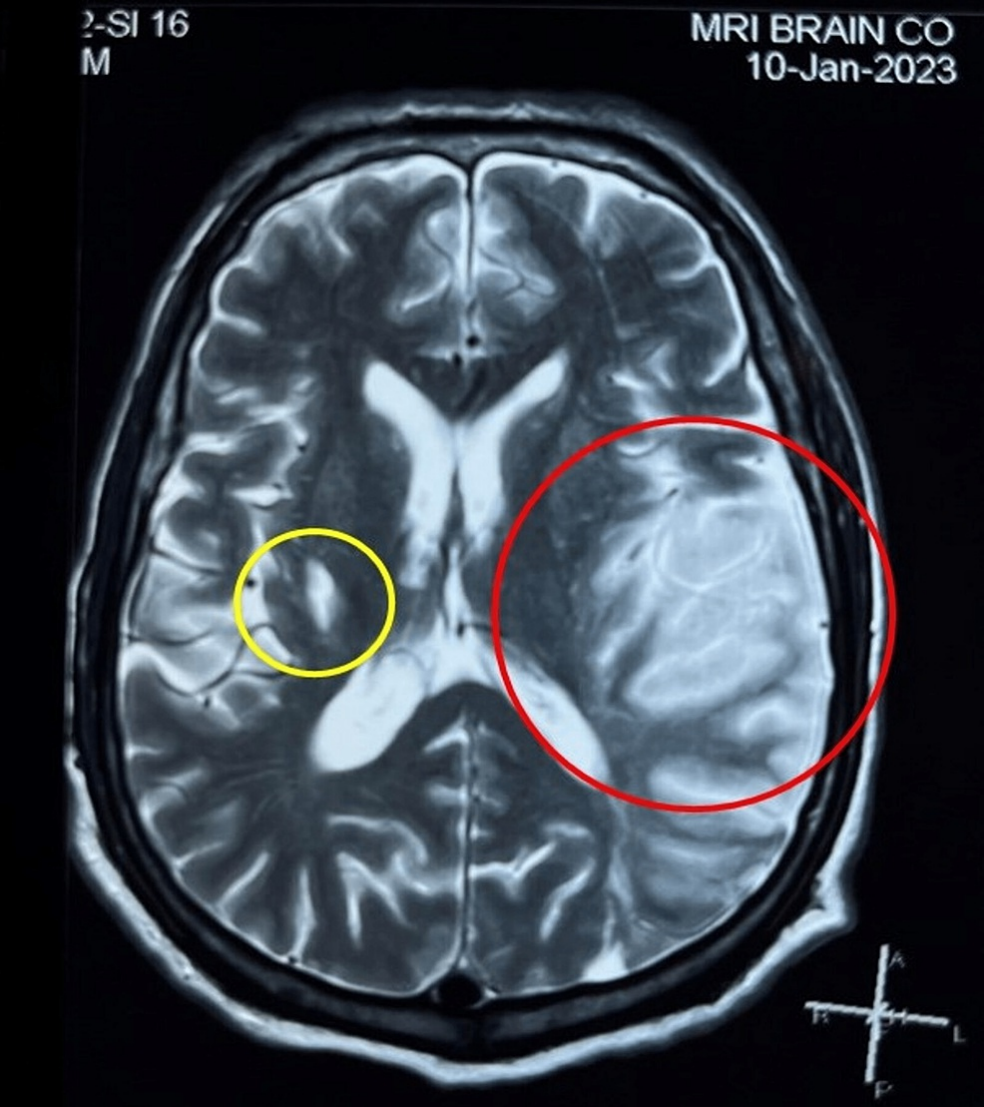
T2-weighted MRI Stroke protocol of the patient shows evidence of acute stroke of the left middle cerebral artery (MCA) territory with chronic infarct in the right ganglion. Red circle indicates acute infarct. Yellow circle indicates chronic infarct.
